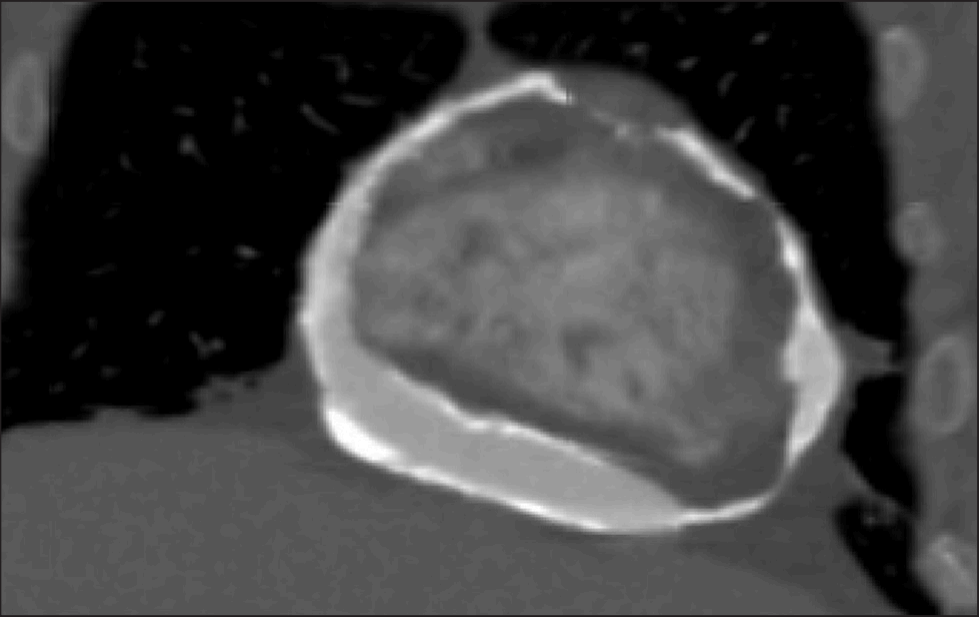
Coronal view of severely calcified pericardium on computed tomography.
